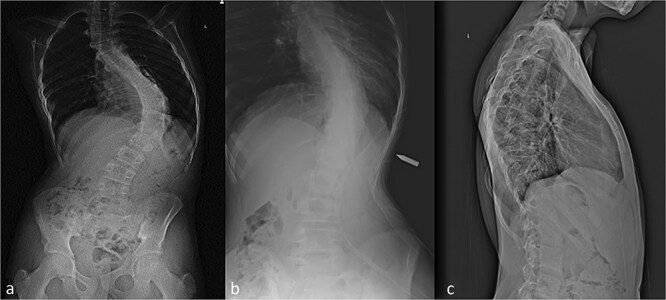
Preoperative whole spine radiographs. AP (a), bolster (b), and lateral (c) views showing 86° thoracic scoliosis with left convexity.
